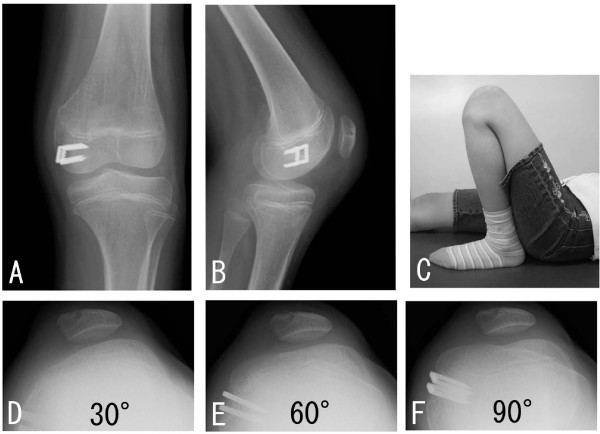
Images taken during surgery and at 2 years follow-up. The reconstructed ligament is fixed just distal to the epiphyseal line as well as the adductor tubercle, and just distal to the posterior aspect of the medial epicondyle (Fig. 5-A, B). Two years after the surgery, the ROM is full (Fig. 5-C), and the plain X-ray demonstrates that the patella is consistently in position at 30°, 60°, and 90° in flexion (Fig. 5-D, E, F).
